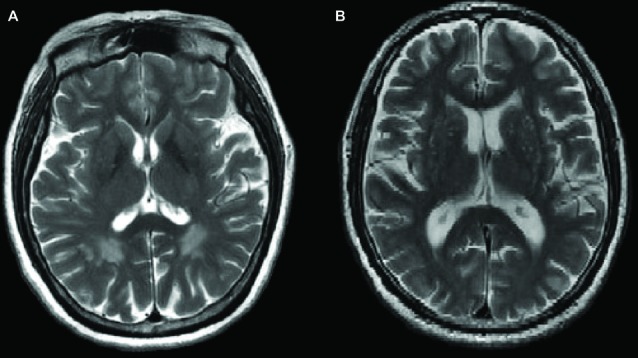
Two typical T2-weighted Magnetic resonance imaging (MRI) images obtained from a occipital periventricular hyperintensities (OPVHs) patient (A) and a healthy control (CN) (B). White matter hyperintensities (WMHs) were bright with higher signal intensities (A). Note that subjects had cerebral infarcts, defined as focal hyperintensities on T2 images.
